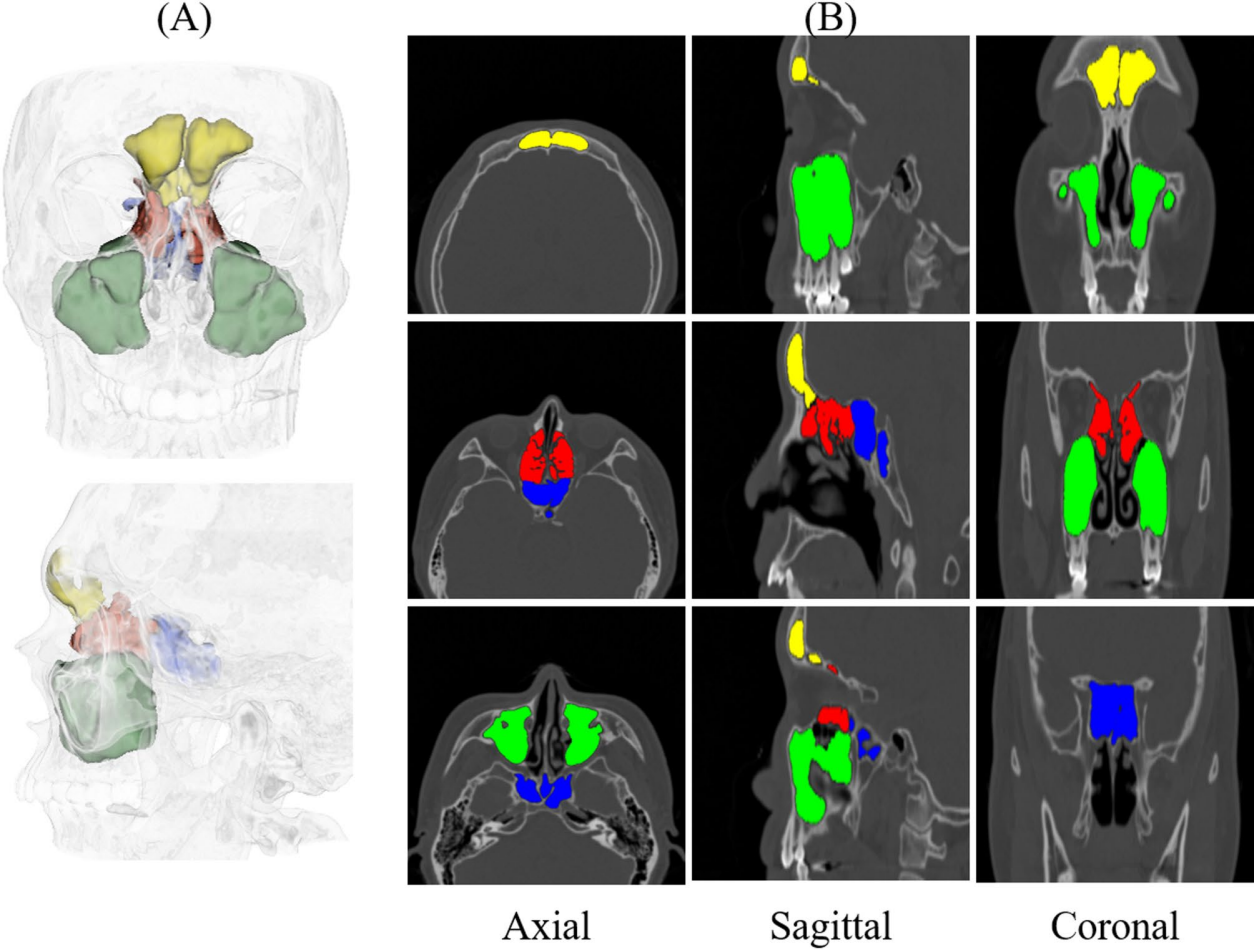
(A) Three-dimensional visualization of the paranasal sinuses consisting of the frontal sinus (FS), ethmoid sinus (ES), sphenoid sinus (SS), and maxillary sinus (MS) regions, represented in yellow, red, blue, and green colors, respectively. (B) A two-dimensional visualization of the sinuses in axial, sagittal, and coronal views with binary masks delineating each sinus in the CT image. (Created with 3D Slicer, 5.6.1, https://www.slicer.org/ ).
